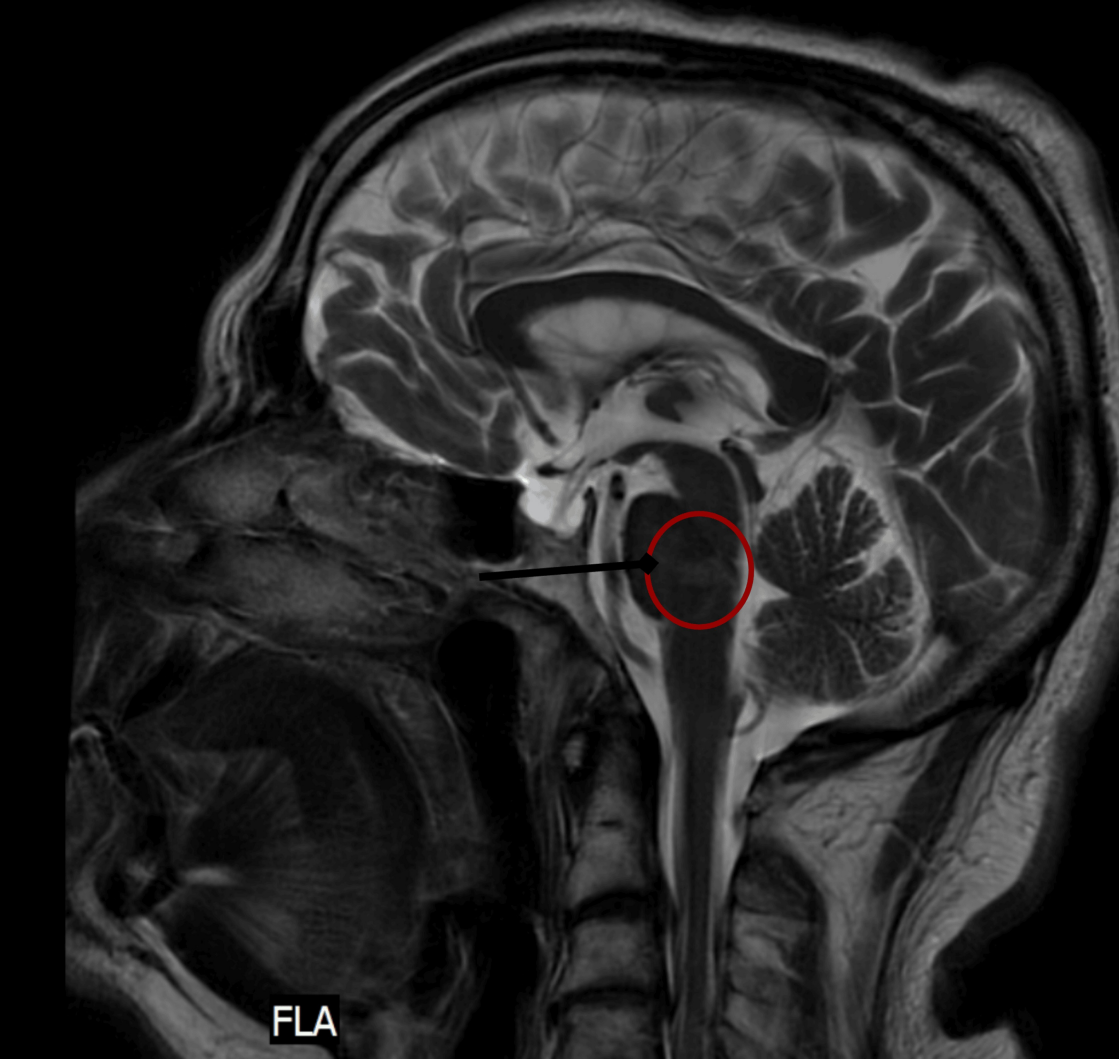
Sagittal view of T2 brain MRI revealed para-sagittal, non-enhancing, restricted hyperintense focus in the posterior aspect of the left side of the pons, indicating the early stage of a subacute infarct MRI: magnetic resonance imaging
